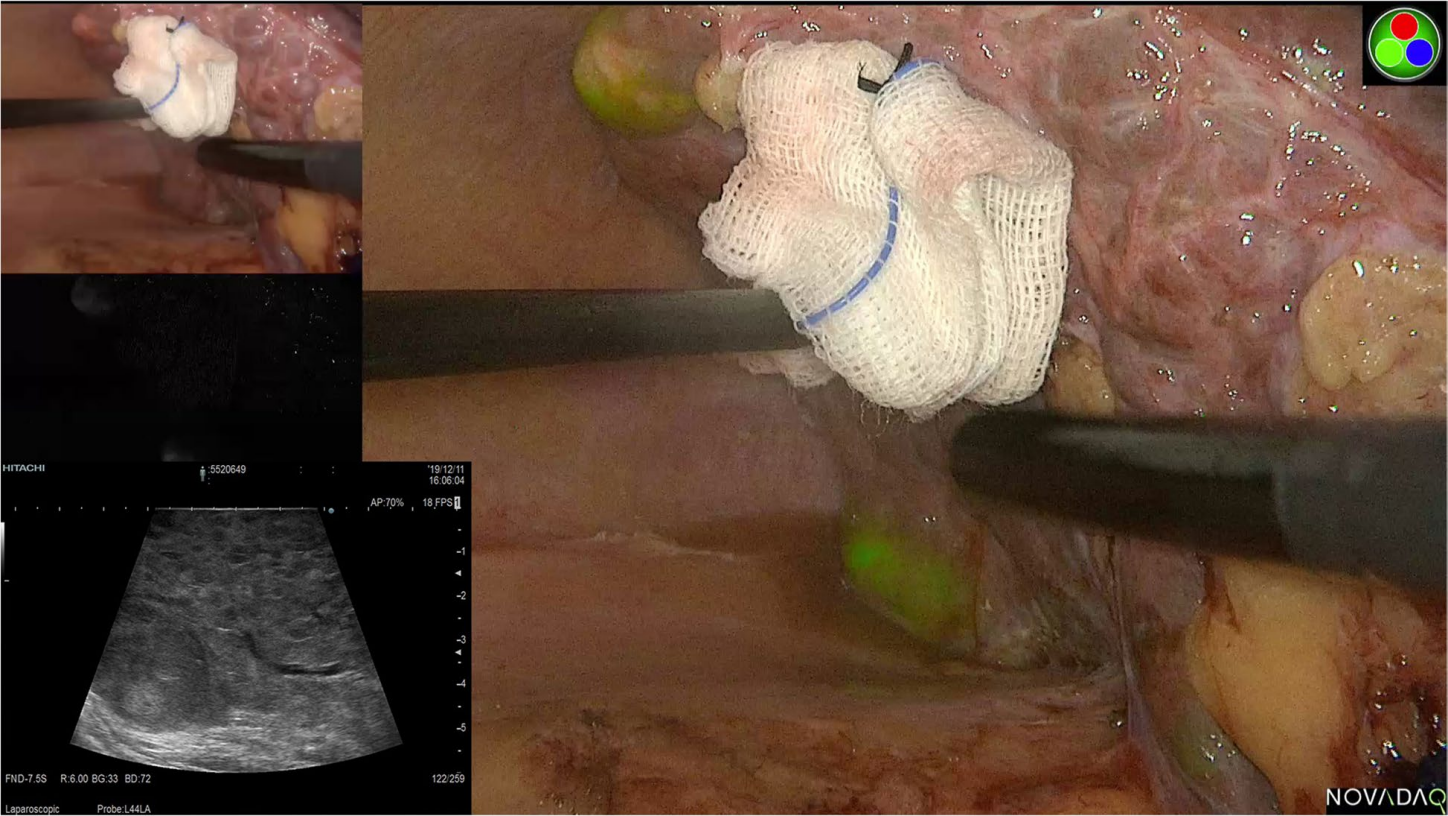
Laparoscopic ultrasound (LUS) combined with ICG fluorescence navigation to improve the intraoperative identification and demarcation of recurrent tumors
